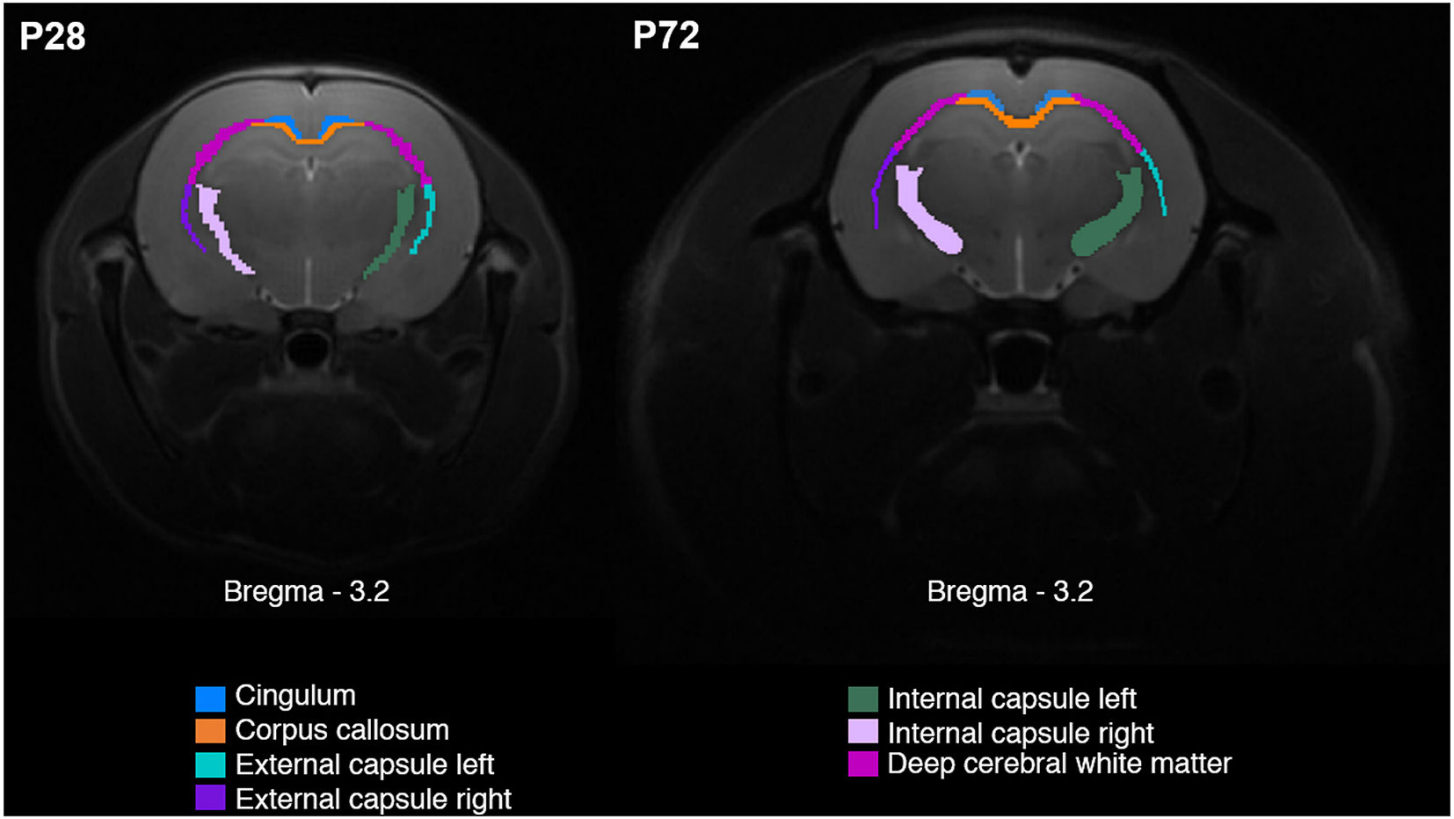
Regions of interest (ROIs) representing white matter bundles. ROIs located at coronal plane −3.2 mm from the bregma used for the analysis of white matter integrity in MRI parametric maps (MTR, T_1sat_, RAFF4, FA, AD, MD, and RD) on postnatal days 28 and 72. ROIs are overlaid on the structural T_2_ relaxation reference brain.
